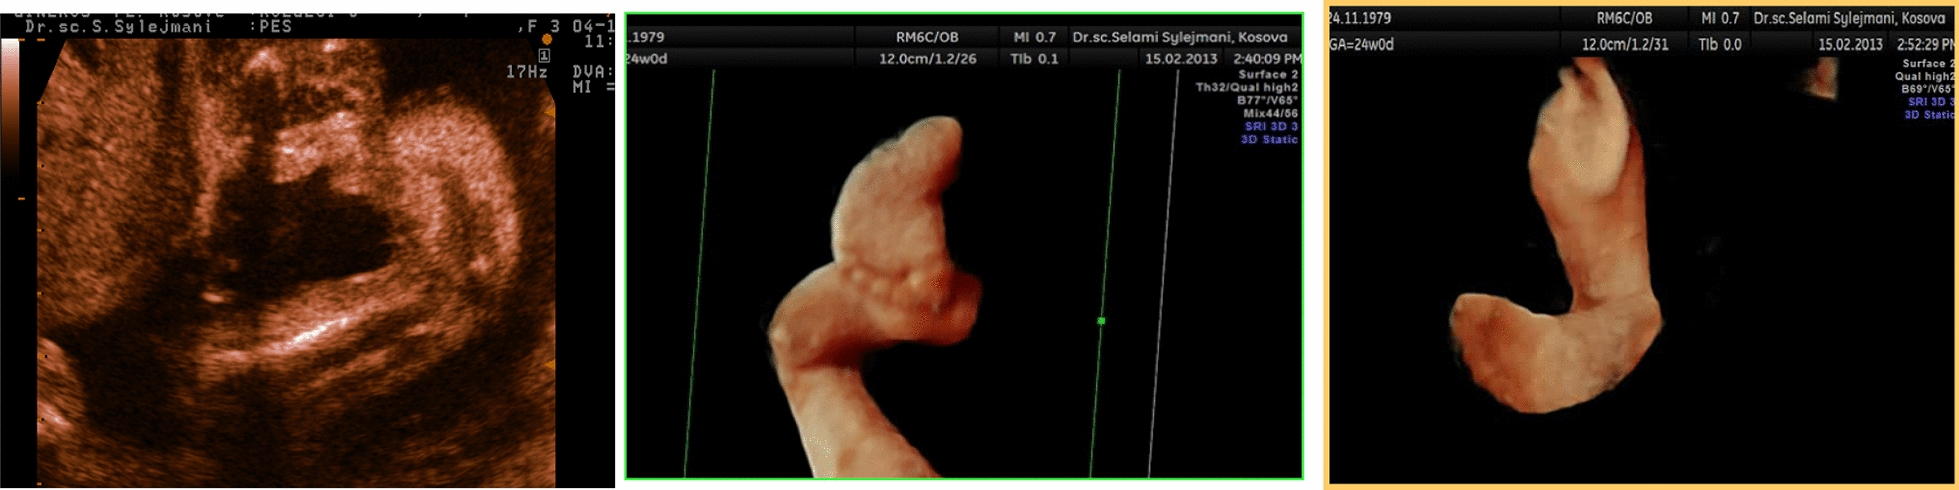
Ultrasound two- and three-dimensional images of clubfoot at 18 weeks of gestation
